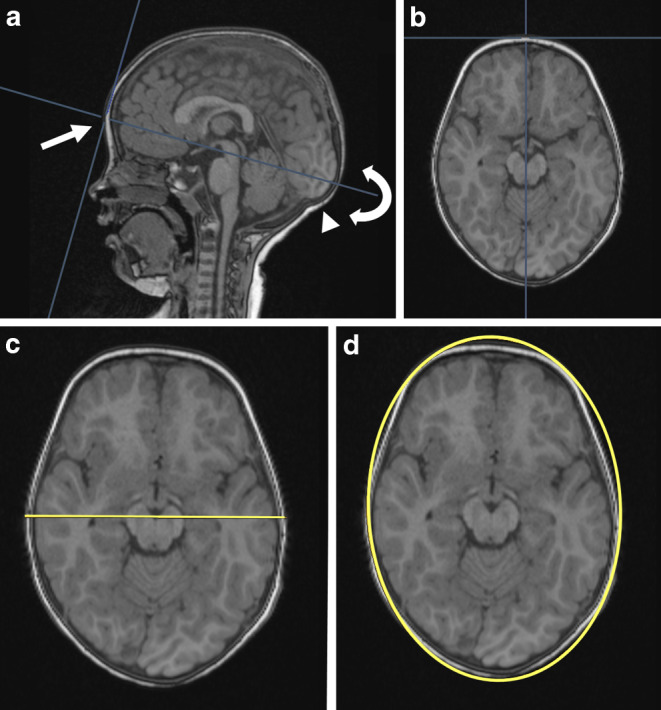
A 2‑year-old girl with microcephaly (clinical head circumference 45 cm/ < 1st percentile) a Midsagittal MPRAGE (Magnetization Prepared – RApid Gradient Echo) 3D-reformat with a nearly normal craniofacial ratio. For MRI-based head circumference estimation identify the supraorbital bulge (*white arrow*). Then adapt the axial plane in the 3D-reformation (depicted by the *white curved arrow*) until the largest supra-auricular head circumference is achieved (as depicted in b). The inion (*white arrowhead*) can be used as a landmark. c, d An ovoid ROI is created in axial reformat by first identifying the lateral expansion (*yellow line* in c) and then the anteroposterior expansion (d). MRI-based head circumference is 45.1 cm
